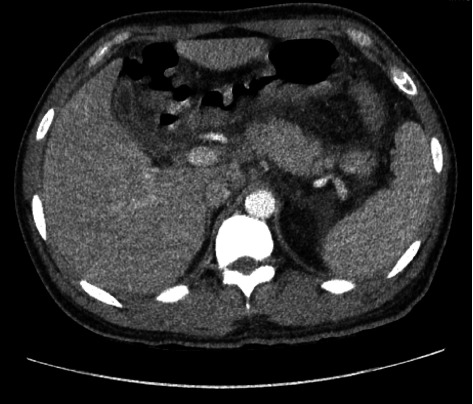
CT Abdomen/pelvis. Diffuse mesenteric edema and fat stranding seen throughout the visualized upper abdomen which could be reactive in the setting of active hepatitis infection.
